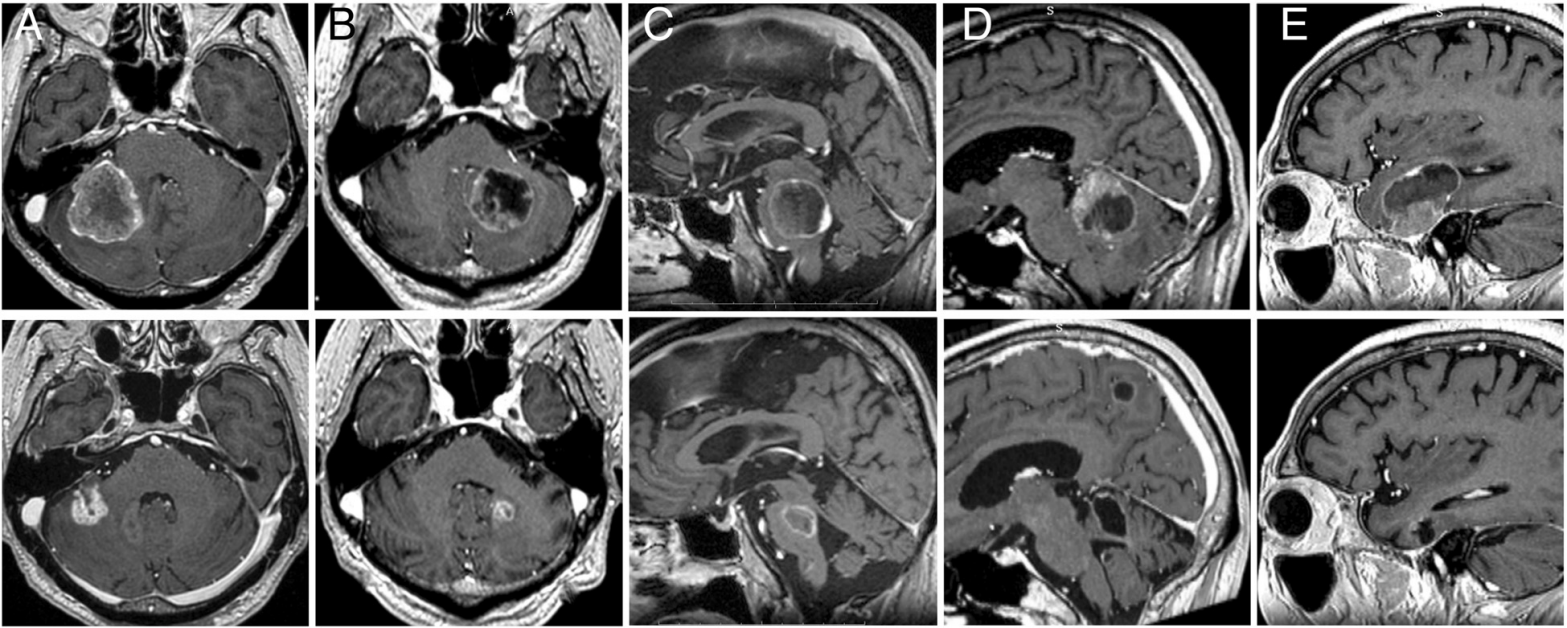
Contrast-enhanced T1-weighted MR images from 5 patients treated with 2-session SRS. The upper and lower images were obtained at the time of the initial SRS and the latest follow-up, respectively. A 71-year-old man with a tumor 19.2 mL in volume *(upper),* 3 months post-treatment *(lower)*: (A). A 60-year-old man with a tumor 14.3 mL in volume *(upper)*, 11 months post-treatment *(lower)*: (B). A 64-year-old man with a tumor 15.2 mL in volume *(upper)*, 8 months post-treatment *(lower)*: (C). A 61-year-old man with a tumor 17.0 mL in volume *(upper)*, 14 months post-treatment *(lower)*: (D). An 80-year-old woman with a tumor 22.3 mL in volume *(upper)*, 9 months post-treatment *(lower)*: (E).
